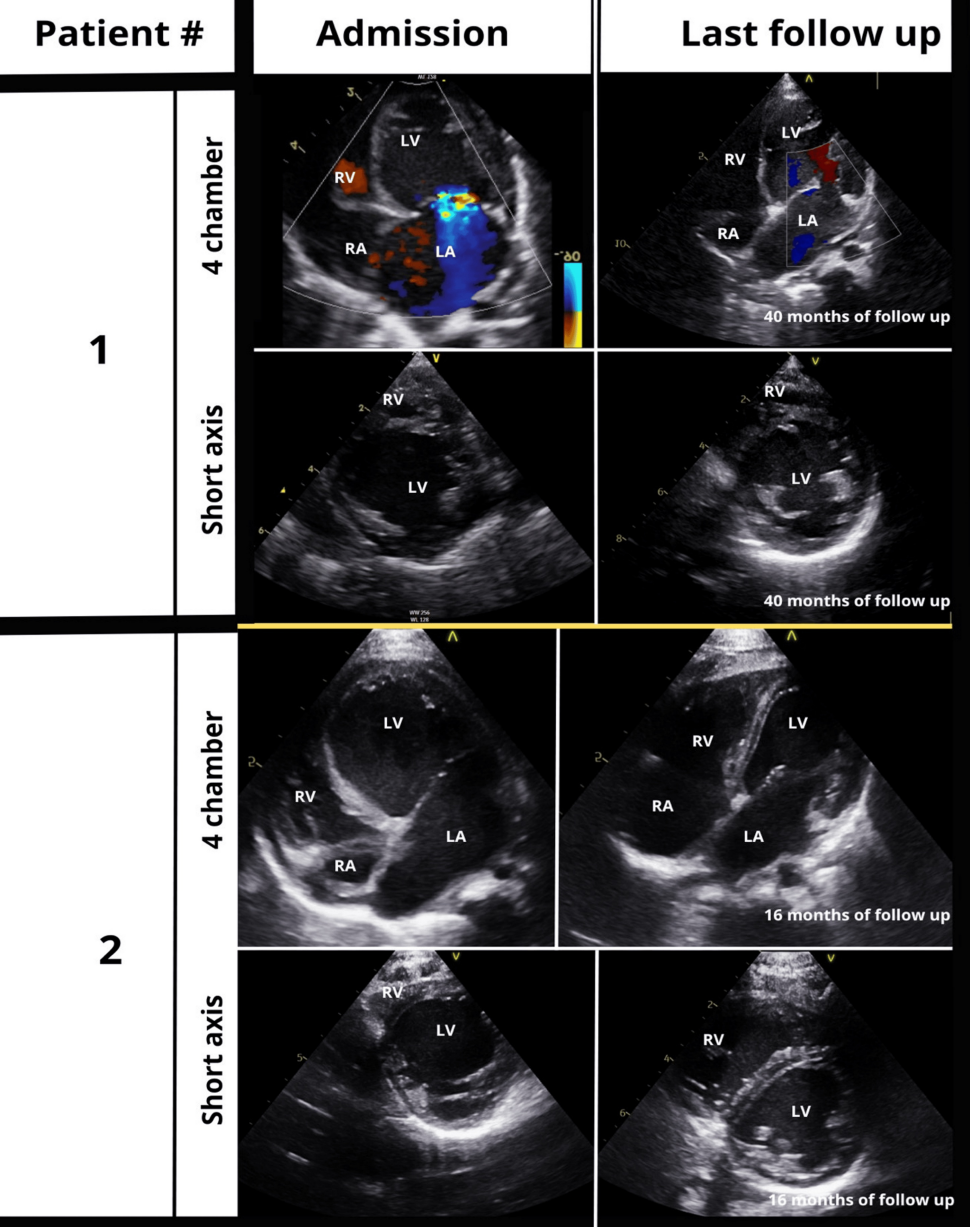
End-diastolic images from four-chamber and short parasternal axis views of patients #1 (Case 1) and #2 (Case 2), acquired at admission and the last follow-up. The gradual improvement of left atrium (LA) and left ventricle (LV) dilation is highlighted. In patient #1, a color Doppler signal was added to show the important mitral valve regurgitation at admission, graded 3/ 4 with a jet area >40% of the left atrium. At 40 months of follow-up, the mitral regurgitation was trivial. The volumes of the right atrium (RA) and right ventricle (RV) were normal in both patients.
